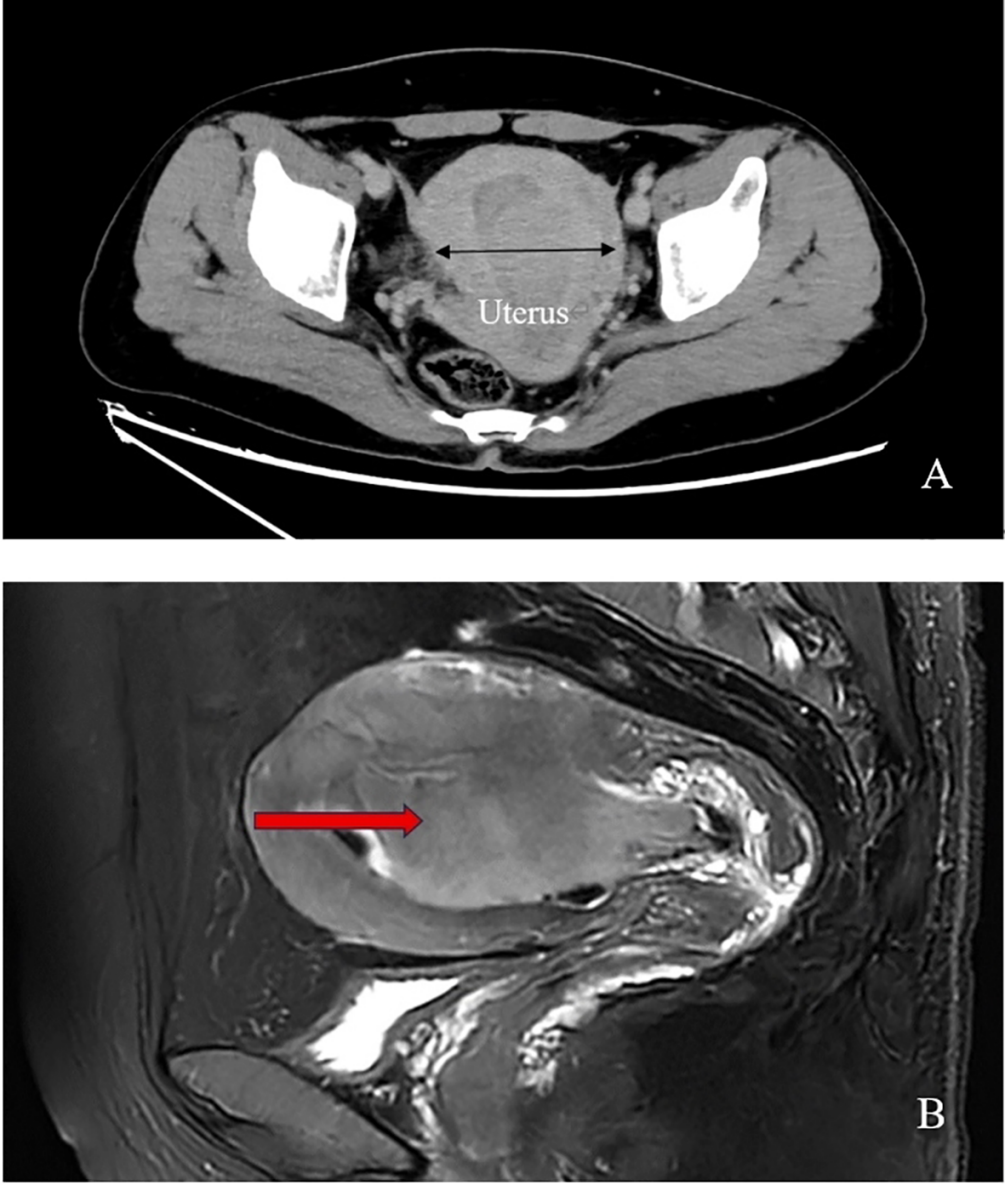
(A) Pelvic enhanced computed tomography scan of the patient shows increased uterine size and a protruding oval-shaped mass in the uterine cavity; (B) Pelvic magnetic resonance imaging reveals an anteverted and bulky uterus with a large oval-shaped mass (arrow point) occupying the entire uterine cavity, resulting in blur endothelium.
